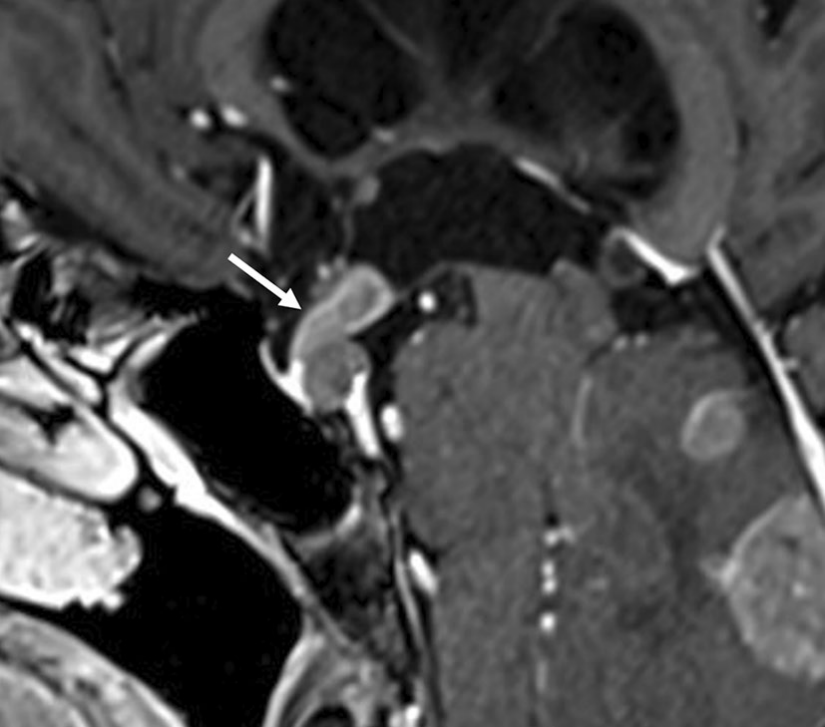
Pituitary metastasis in a patient with breast cancer. A sagittal contrast-enhanced 3D fat-suppressed T1-weighted image shows a dumbbell-shaped pituitary and stalk mass (arrow). Cerebellar metastases and a narrowed fourth ventricle are also visible
